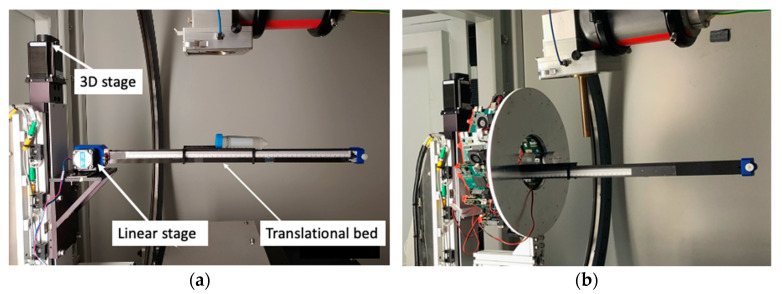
Photos of (a) the translational bed driven by a linear stage and (b) the integrated PET/CT for dual modality imaging.
